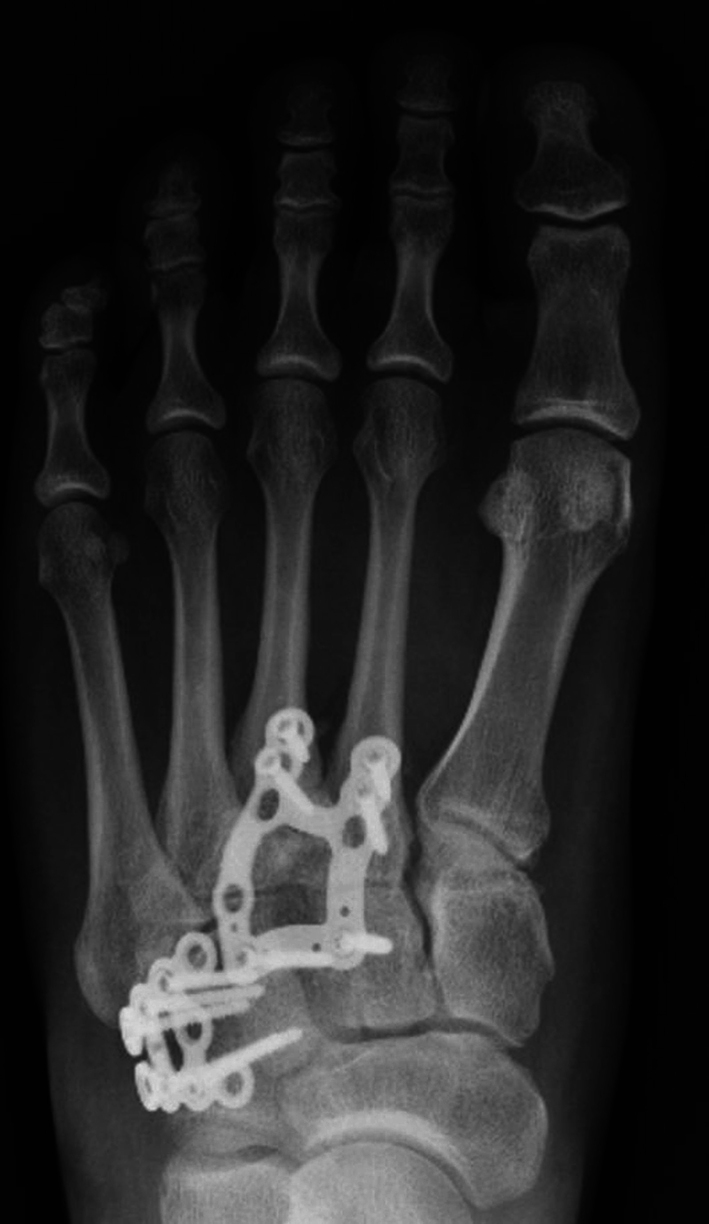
Anteroposterior radiograph of the patient in Fig. 1, treated with a central column bridge plate and cuboid buttress plate
